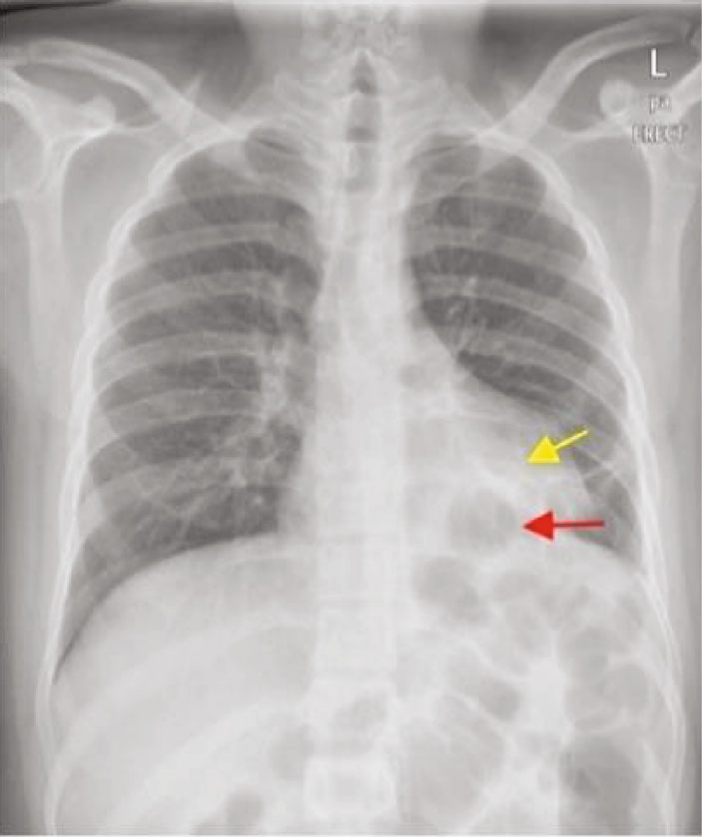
PA chest radiograph shows a left lower zone heterogeneous opacity silhouetting the left hemidiaphragm (yellow arrow) and a retrocardiac air lucency (red arrow).
